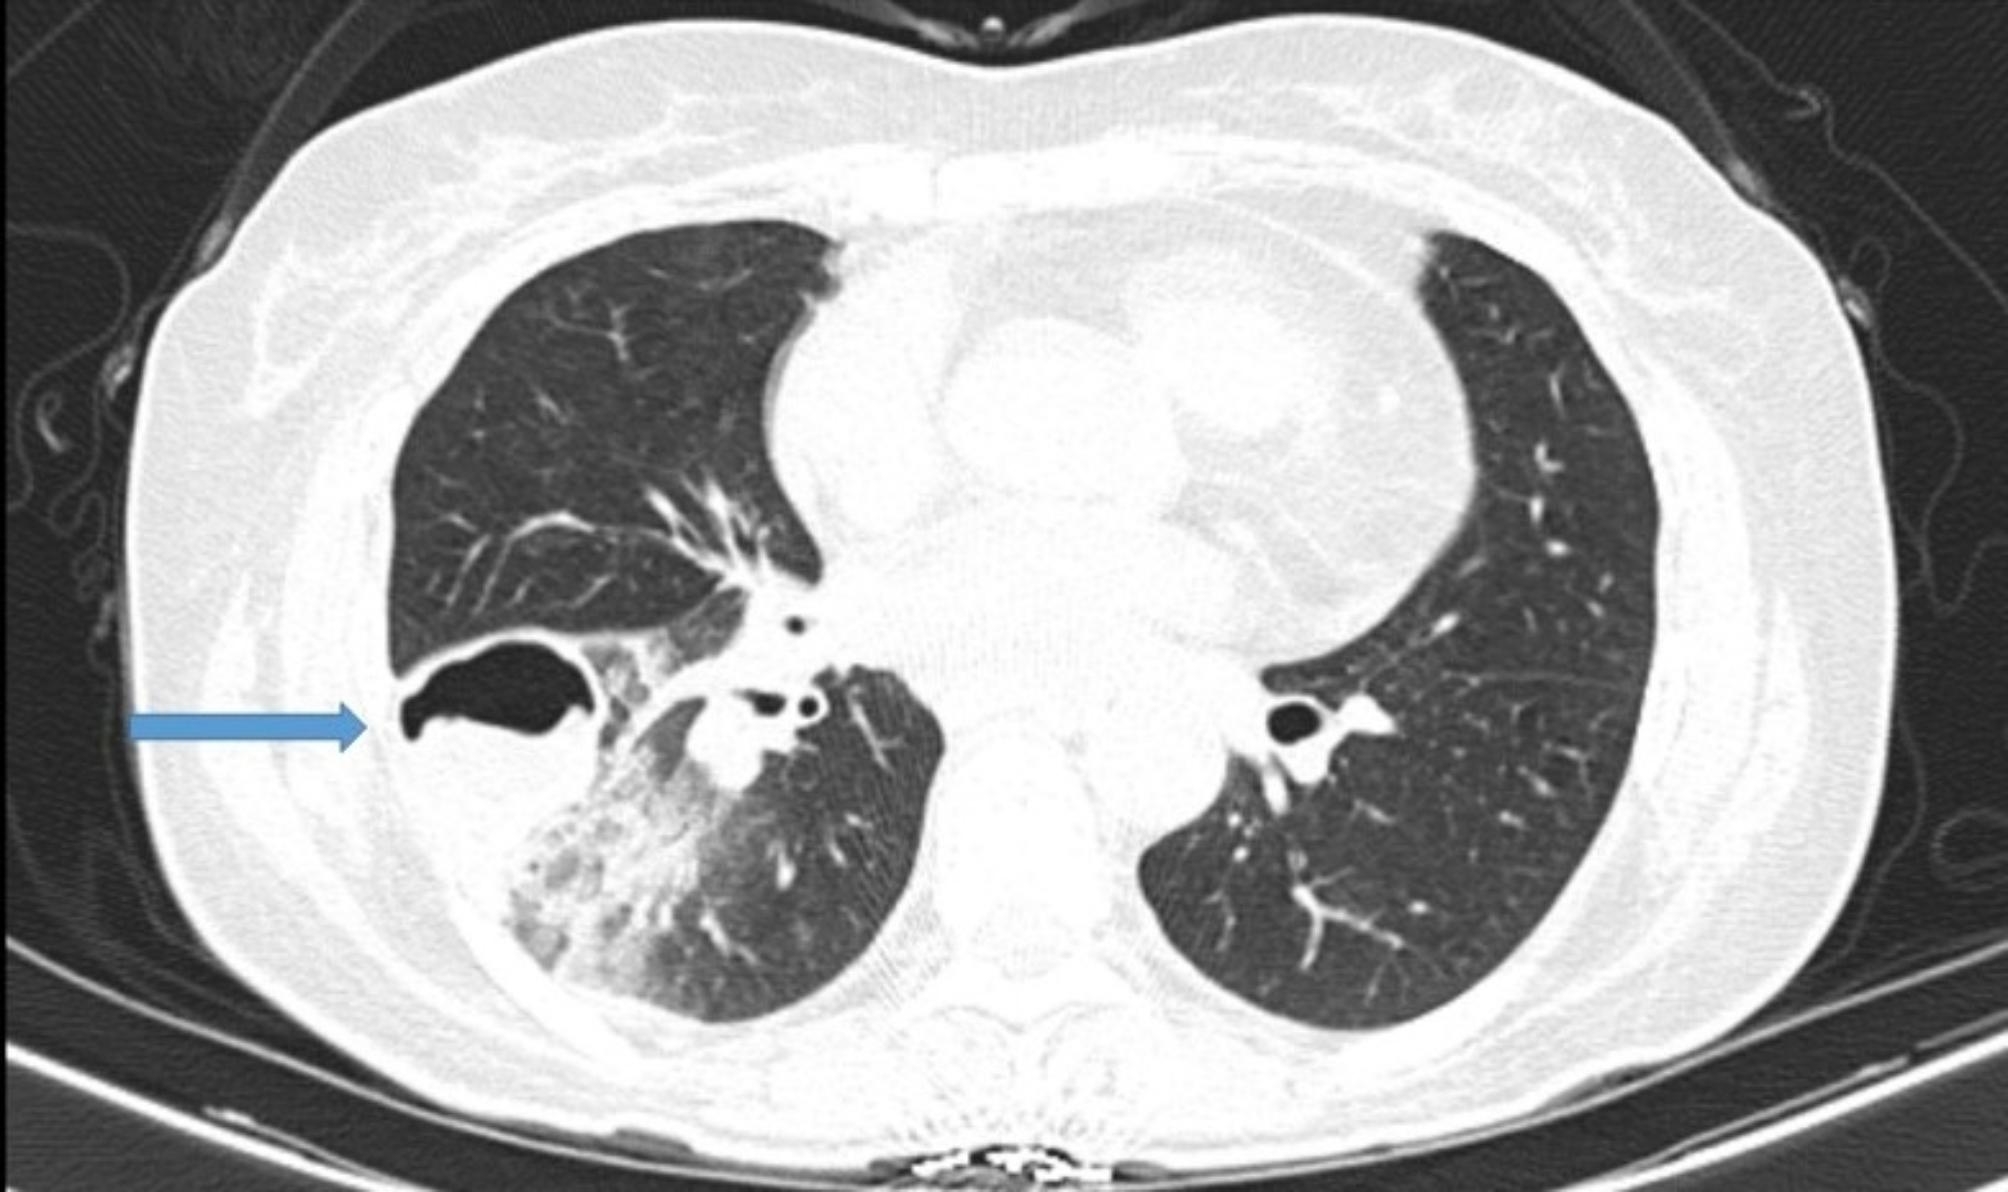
Lung CT scan shows a cavity with thick, regular walls and undulating air-fluid levels measuring 43 × 30, evident in the upper segment of the RLL (blue arrow)
